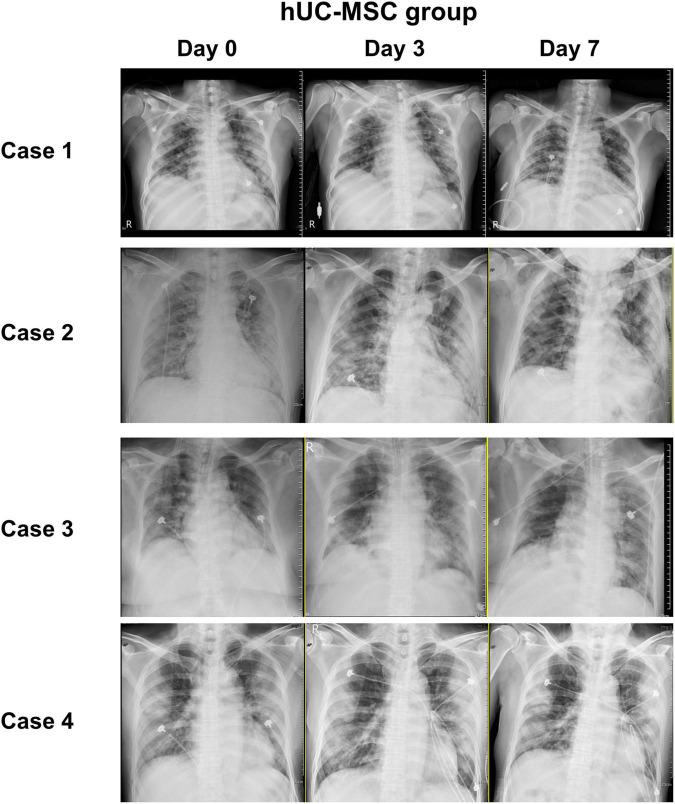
Chest image records of hUC-MSC patients. Serial chest X-ray films obtained on admission day, post-hUC-MSC day 3, and post-hUC-MSC day 7 showed progressive improvements on pulmonary infiltrations in all patients receiving hUC-MSC infusions. Abbreviations as in Figure 2.
